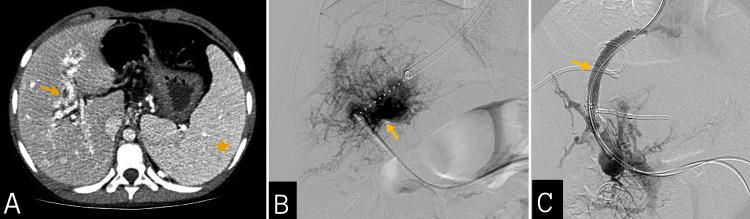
Abdominal contrast-enhanced computed tomography and portographies (A) Abdominal contrast-enhanced computed tomography shows the cavernous transformation of the portal vein (yellow arrow) and splenomegaly (yellow star). (B) Trans splenic portography shows the cavernous transformation of the portal vein (yellow arrow). The puncture was made from the middle hepatic vein to the portal circulation (white dots) using a hydrophilic vascular catheter in the portal cavernoma (yellow arrow) as a guide. (C) Successful portosystemic intrahepatic shunt implantation with the Viatorr stent (yellow arrow).
